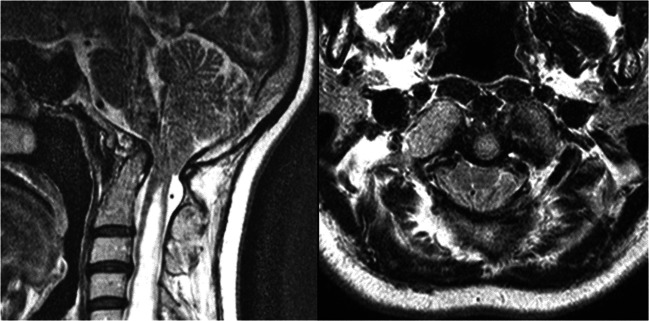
Chiari malformation type I with syringomyelia in a 46-year-old female. Magnetic resonance imaging, sagittal (a) and axial (b) planes
